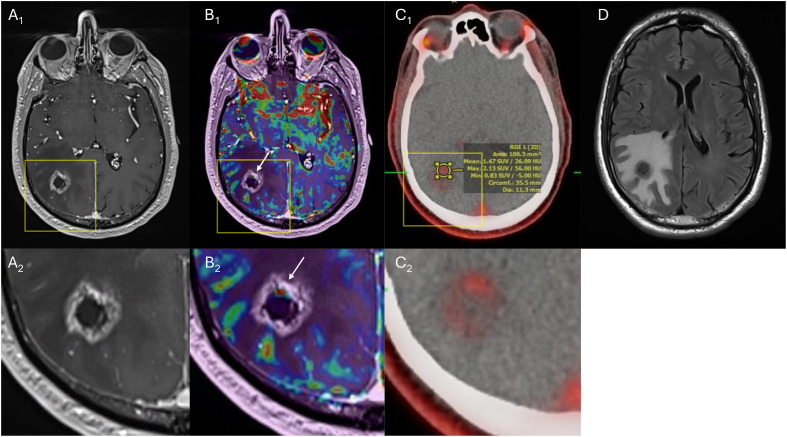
A magnetic resonance imaging (MRI) brain with dynamic susceptibility contrast (DSC) perfusion and an ¹⁸F-fluciclovine positron emission tomography/computed tomography (PET/CT) scan performed approximately 12 months after linac-based stereotactic radiosurgery (SRS). (A_1_) Axial T1c+ demonstrates a 2.3 × 2.1 cm peripherally enhancing lesion; the yellow box denotes the magnified view in (A_2_). (B_1_) DSC perfusion overlay demonstrates increased perfusion within the anterior enhancing components (white arrow); the yellow box denotes the magnified view in (B_2_). (C_1_) An ¹⁸F-fluciclovine PET/CT scan demonstrates low radiotracer uptake (maximum standardized uptake value [SUVmax] 2.13); the yellow box denotes the magnified view in (C_2_). (D) Axial T2 fluid-attenuated inversion recovery (FLAIR) demonstrates increased surrounding edema.
